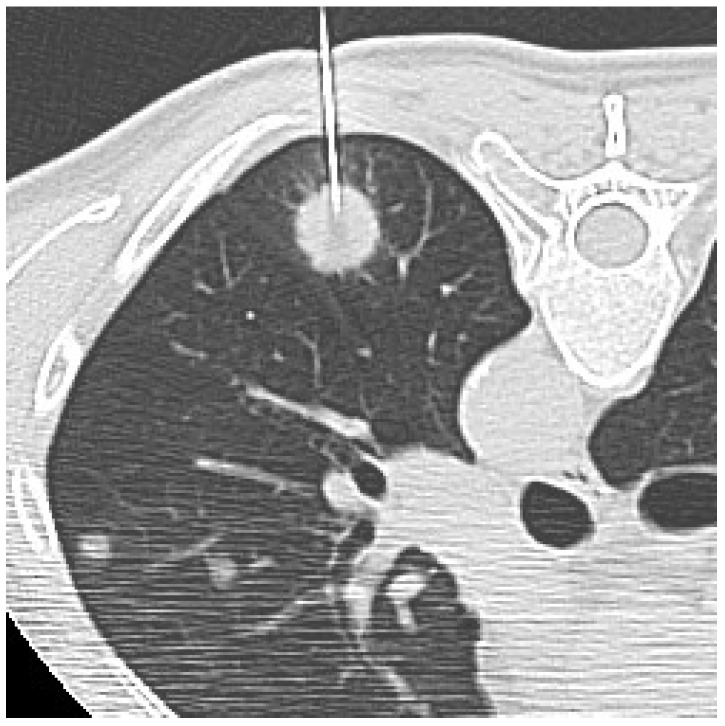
CT-guided biopsy of a solid pulmonary lesion in the left lower lobe. Supine unenhanced CT scan obtained before sampling shows a satisfactory position of the needle tip within the lesion.
